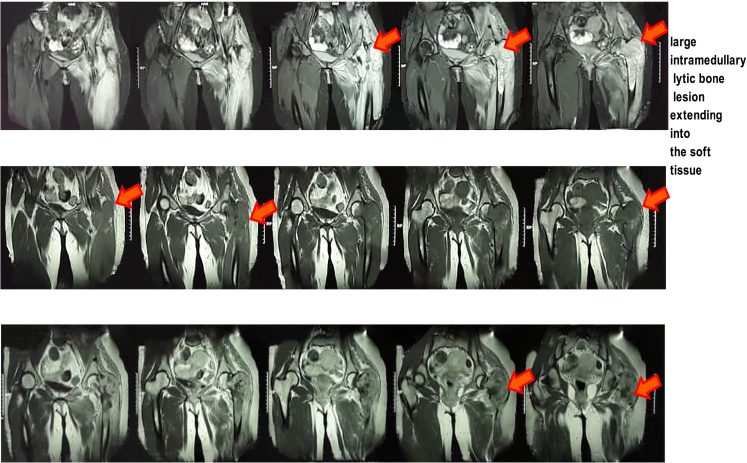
MRI of the pelvis showed a complex irregular border T1/T2 signal intensity, intramedullary lesion. The mass had a 131 × 70 mm × 60 mm size compressing the left piriformis muscle posteriorly as indicated by orange arrows. The tumor had heterogeneous enhancement at diaphysis and metaphysis of proximal part of left femur with disruption of cortex and infiltration to adjacent anterior and posterior muscle compartment. There was no involvement of neurovascular bundle.
